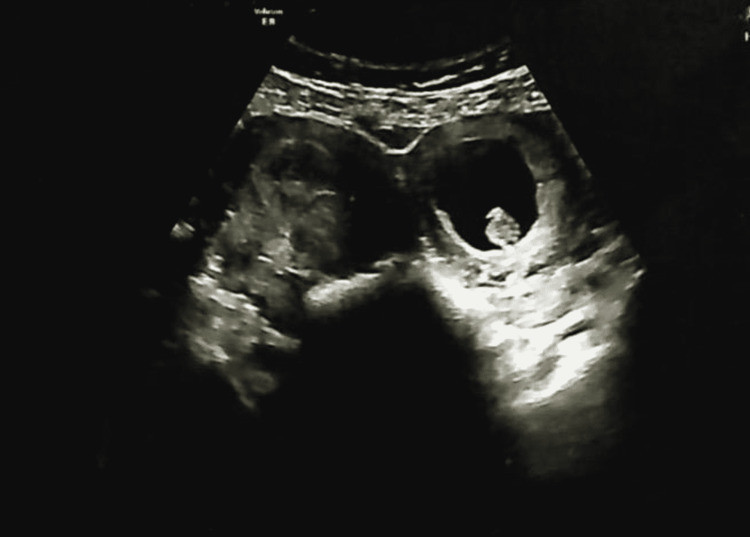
Ultrasound scan showing foetus in the extra uterine space, raising an index of suspicion for an RHP RHP: rudimentary horn pregnancy
